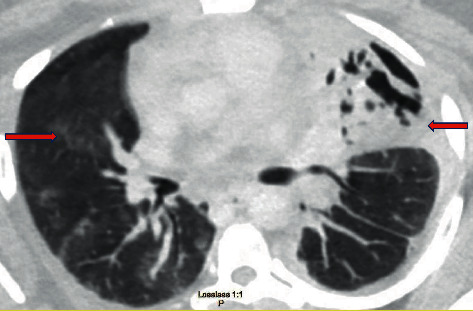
CT chest revealing decrease in left size empyema, interval development of a large focus of necrotizing pneumonia within the right middle lobe, and interval improvement in right lung multifocal pneumonia.
